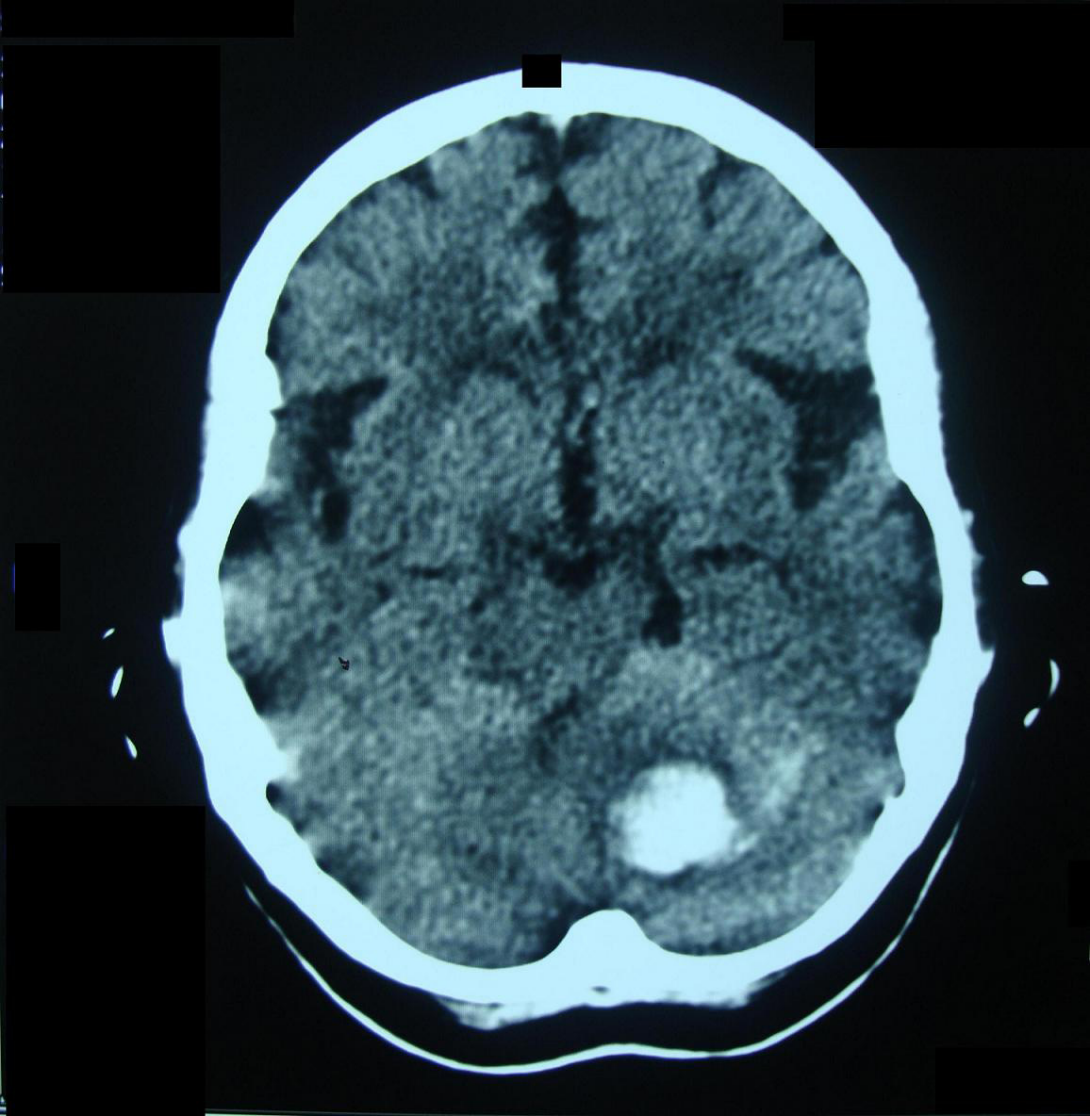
Non-contrast brain CT scan of the same patient, which was done about 2 hours after the re-bleed. The second hemorrhage lies at the left deep dentate nuclei and is not surrounded by edema, implying a very recent development. The first hematoma at the right cerebellar hemisphere had resolved, leaving a small slit.
